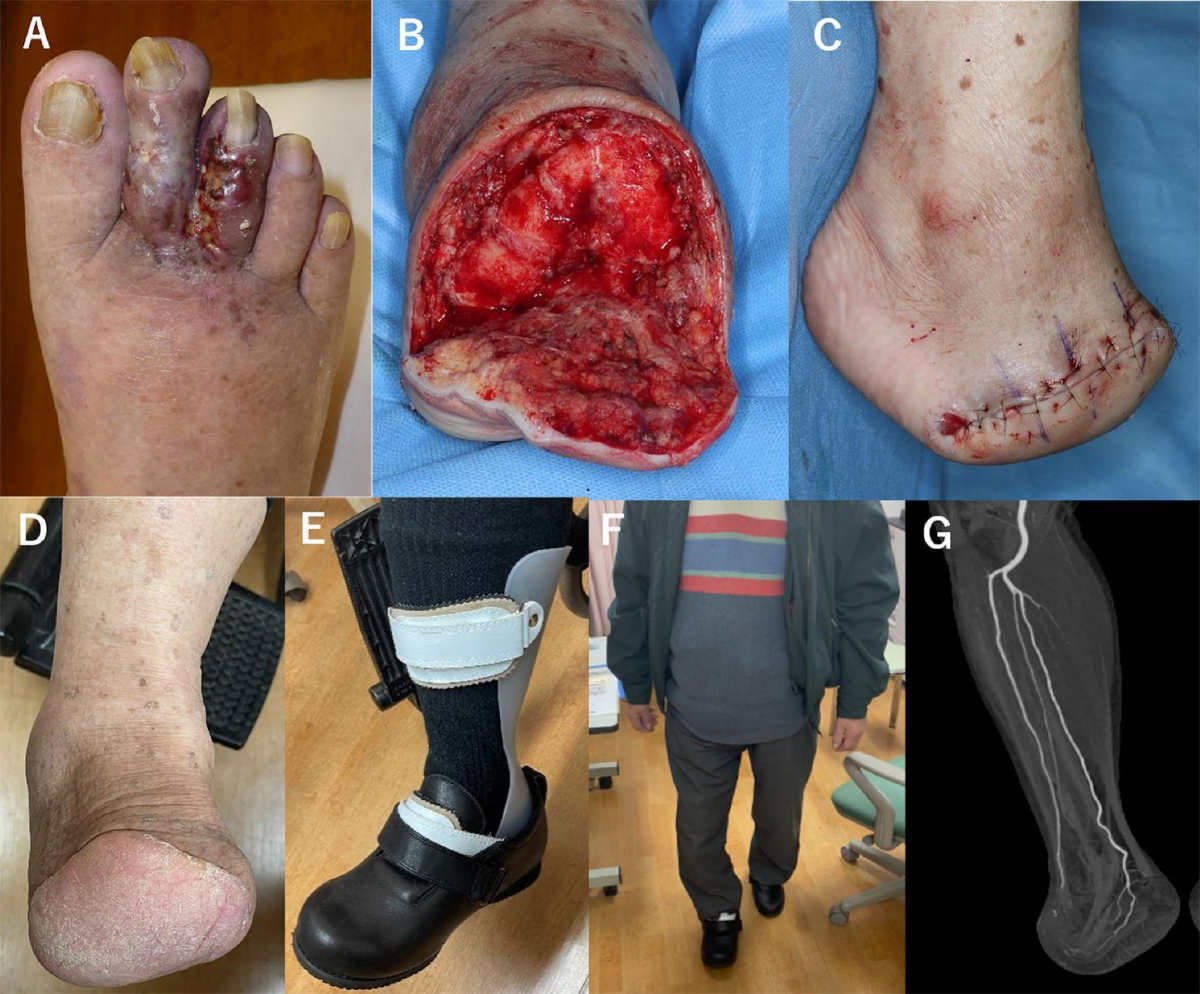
Ulceration and surgical resection of arteriovenous malformation (AVM). Ulcers occurred on the treated right toes after embolization (A). Disarticulation at the Lisfranc joint, including surgical resection of AVM (B, C). Wound healing and ambulation were achieved with assistance equipment (D–F). Postoperative computed tomography angiography showed that AVM was completely resected (G)
